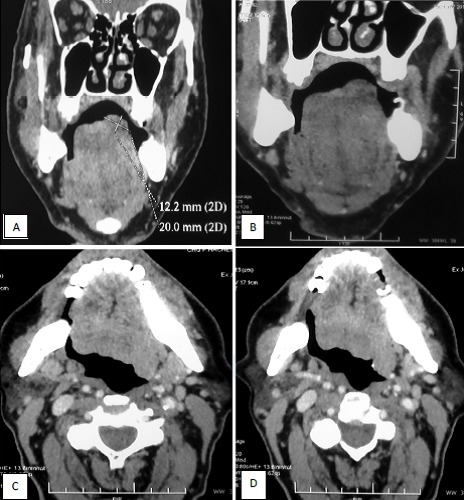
Tissue process of the root of the tongue: coronal (A) and axial (B); contrast-enhanced CT scan showing tissue process of the left margin of the tongue measuring 20x12 mm (C, D)
